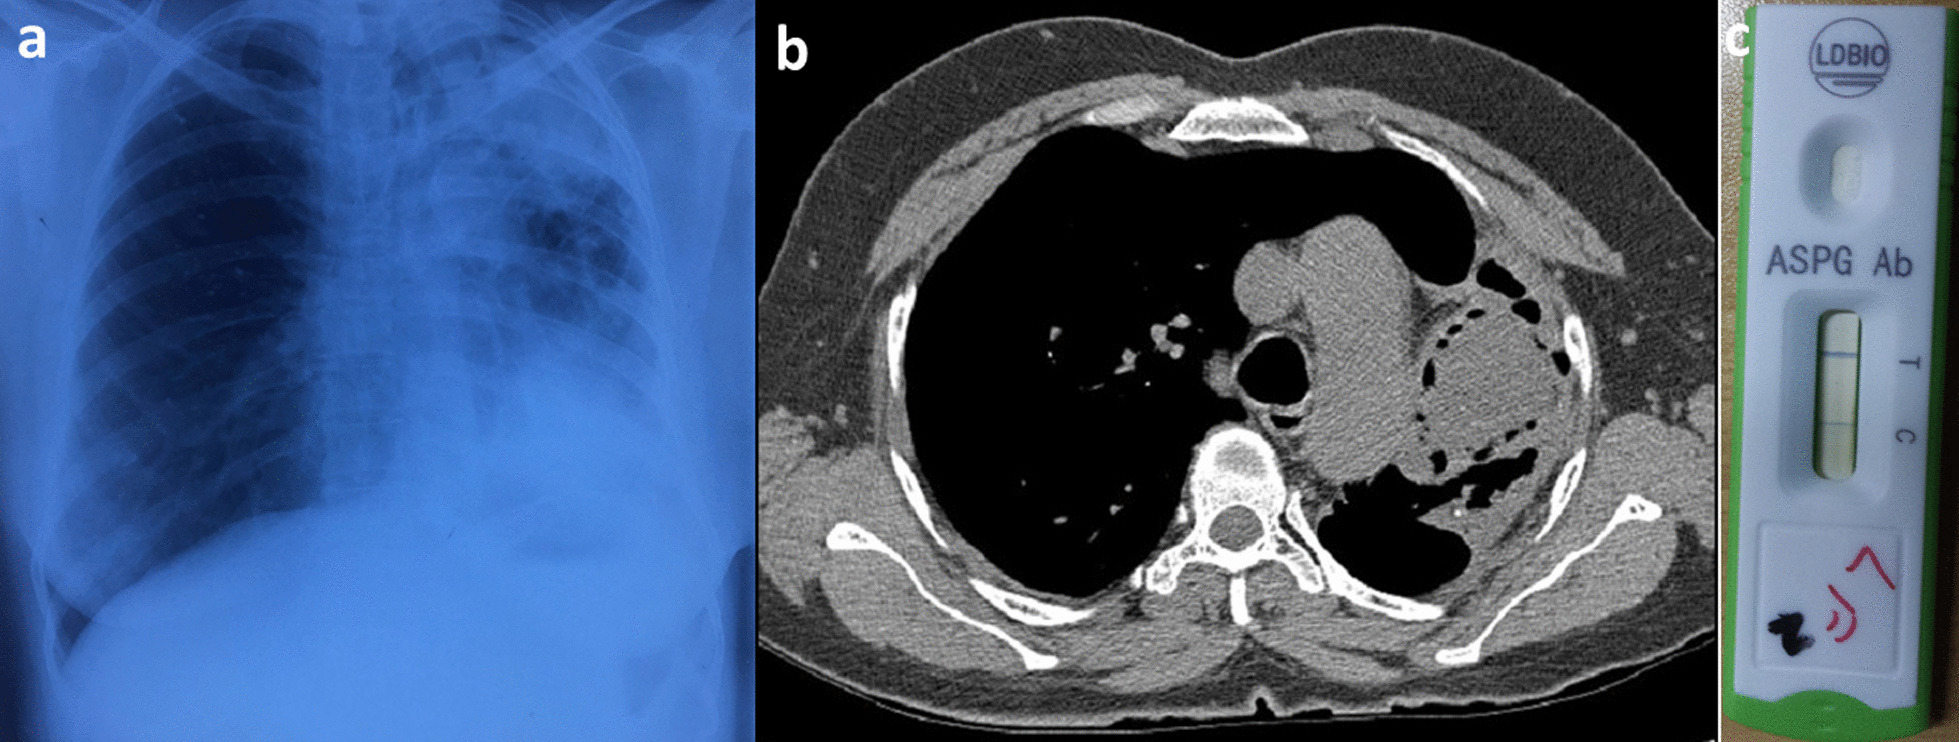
Case 1. a Chest x-ray showing a mass in the left upper lung zones with an “air crescent sign” and fibrotic changes in the lower zones. b Chest CT scan showing an ovoid mass surrounded by pockets of air, most probably a fungal ball with local pleural thickening. c Positive *Aspergillus* IgM-IgG LFD
